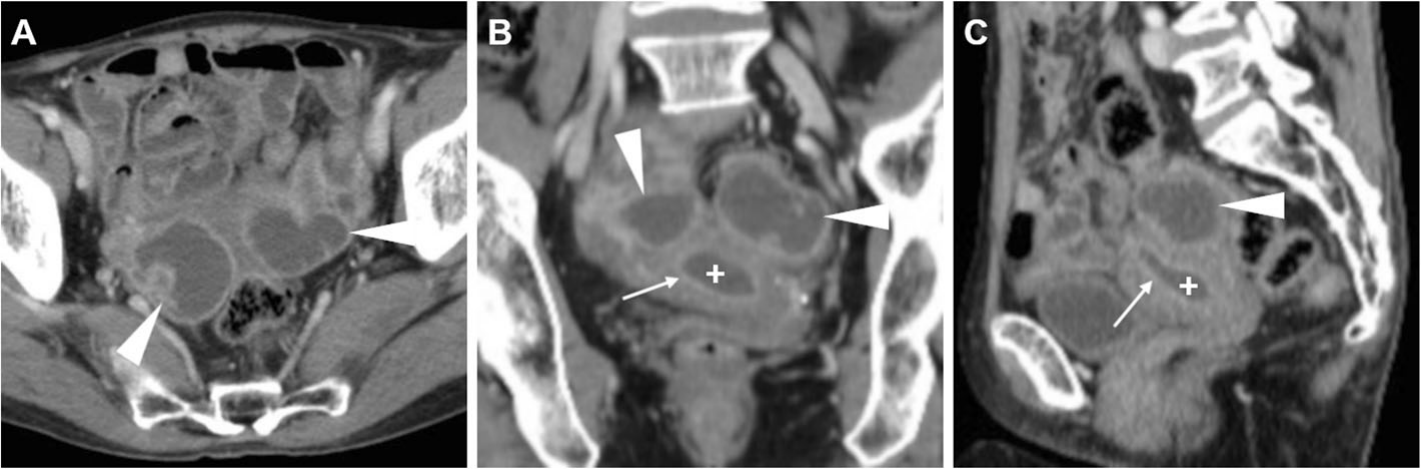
a–c Multiplanar CT images of genital involvement in a 58-year-old woman with respiratory tuberculosis, showing bilateral adnexal abscess-like enlargement (arrowheads) with peripheral enhancement, and dilated uterine cavity with thin endometrial enhancement (thin arrows) suggesting pyometra [adapted from Open Access ref. [50]]
